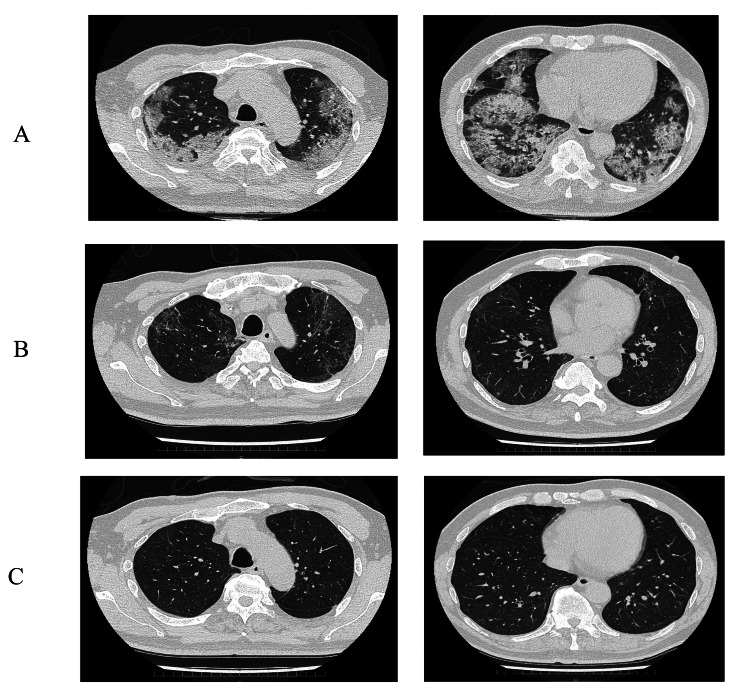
CT scans of the patient at admission (A) and at the one-month (B) and six-month (C) follow-up visits. These images show the time course of the patient's dissolving bilateral lung opacities.
